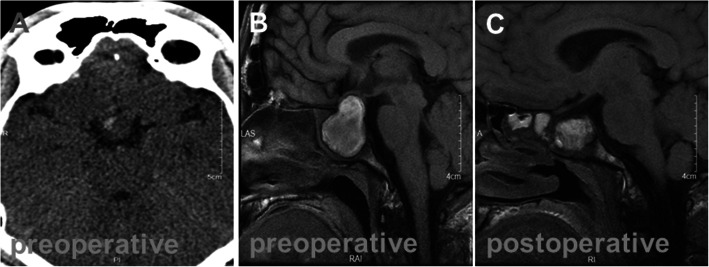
The brain images of the patient were evaluated by CT and MRI. a Preoperative computed tomography revealed pituitary hemorrhage (white arrow). b Preoperative contrast-enhanced T1-weighted magnetic resonance images exhibited pituitary tumor with hemorrhage, lobulated mass measuring 2.6 × 2.7 × 3.2 cm at the sella region with a prominent suprasellar component and vascular encasement. c Postoperative MRI images showed no residual tumor
